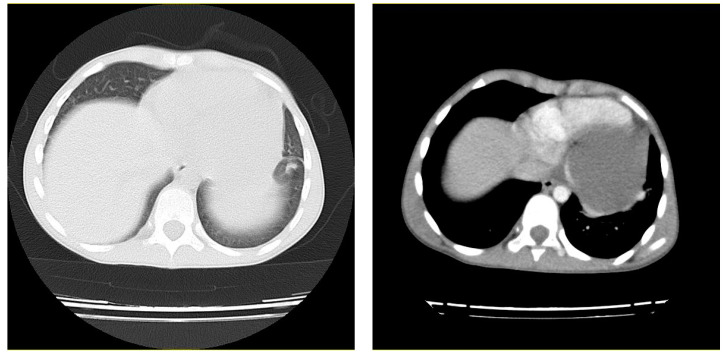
Axial view of the patient’s contrast-enhanced chest computed tomography (CT) scan. A retrosternal mass characterized with a clear serous fluid with compression on the posterior wall of the left ventricle.
